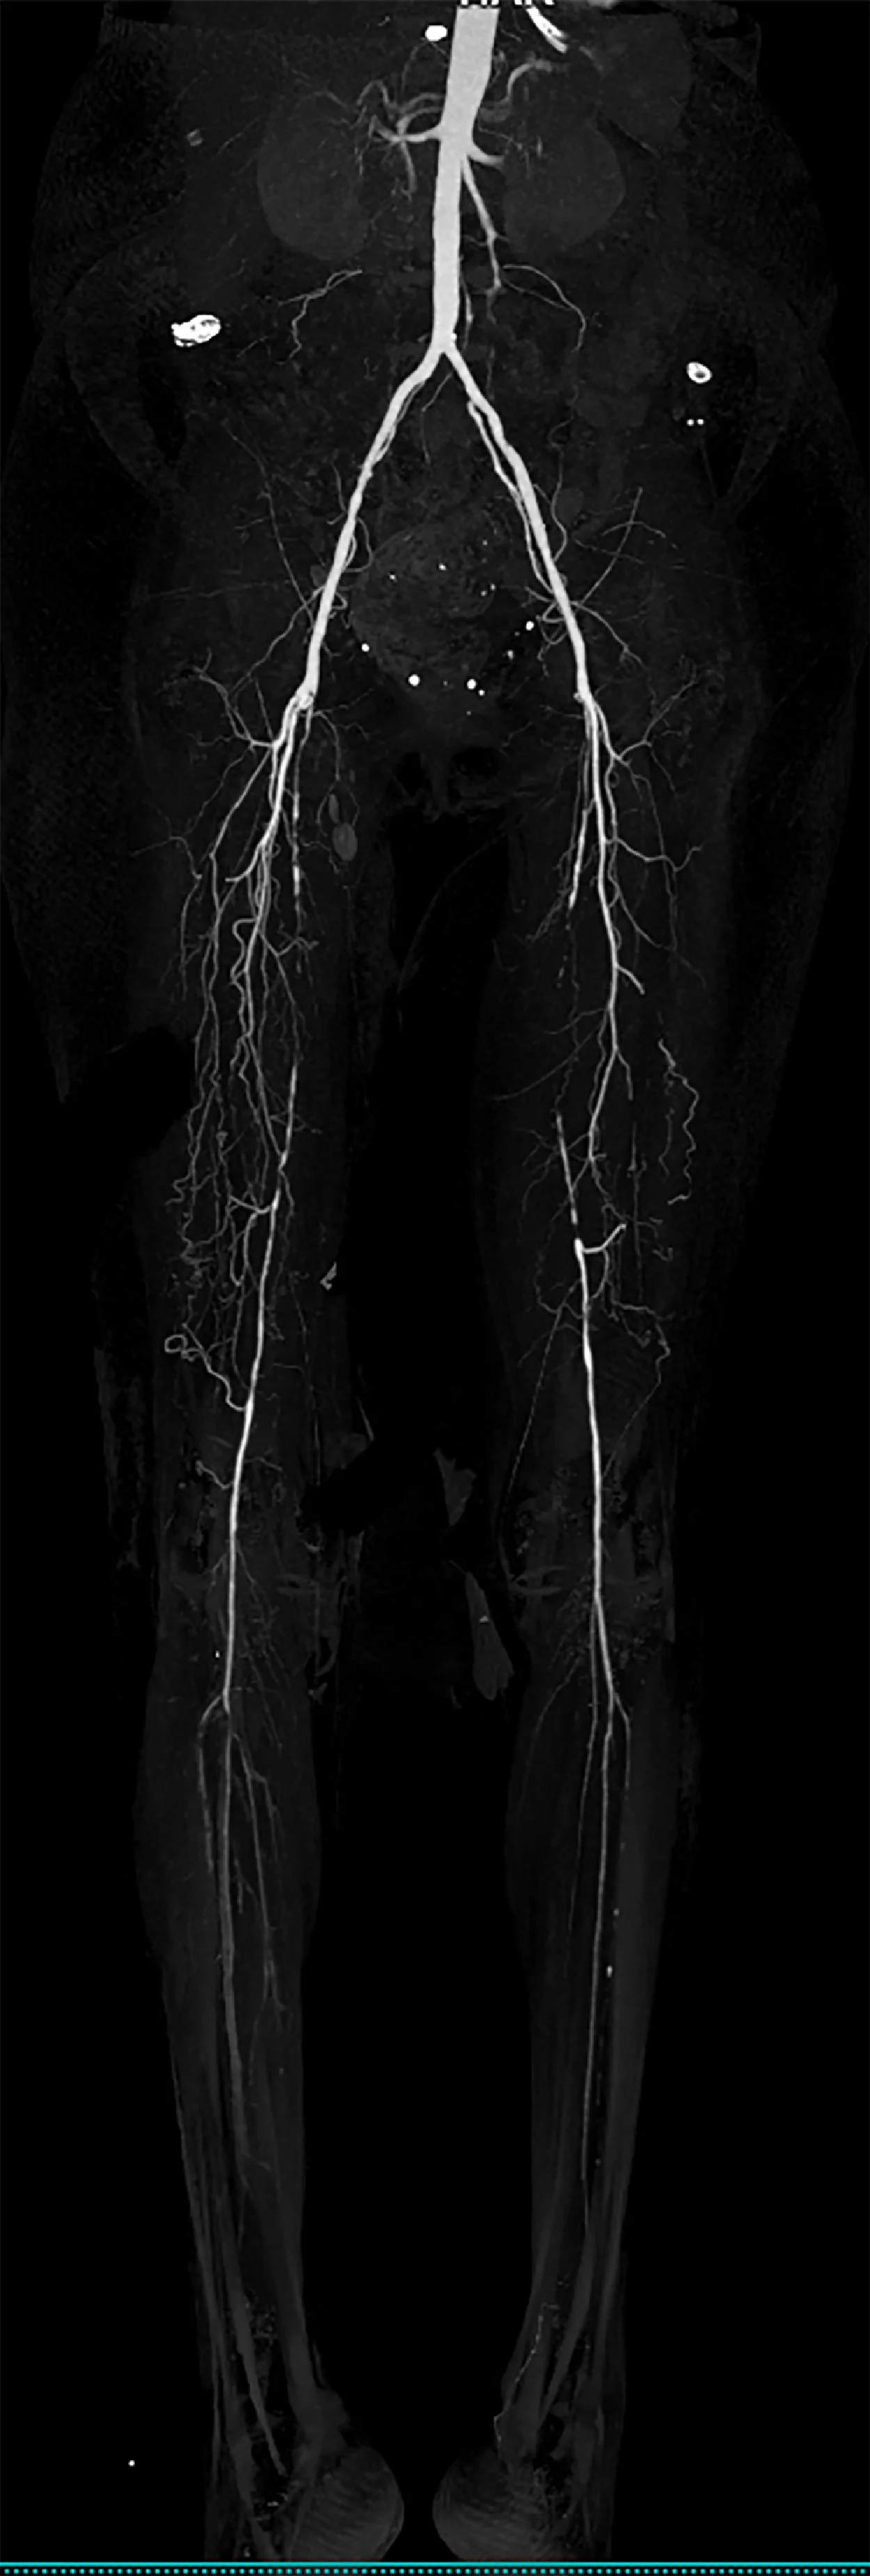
CT angiography of the lower extremities (maximum intensity projection, coronal view). Coronal MIP reconstruction of the aorto-iliac and lower extremity arterial tree demonstrates patent infrarenal aorta with abrupt loss of flow signal at the level of both common femoral arteries, consistent with bilateral femoral artery thrombotic occlusion. Reconstitution of the bilateral superficial femoral arteries is observed, likely via pelvic collateral pathways. Multisegmental irregularity and discontinuity of flow signal are noted along the superficial femoral, popliteal, and crural axes bilaterally, with more pronounced signal attenuation on the right side, consistent with critical limb ischemia. These findings confirm the diagnosis of severe multilevel lower extremity arterial disease (LEAD) in the setting of a systemic arterial thrombotic process.
